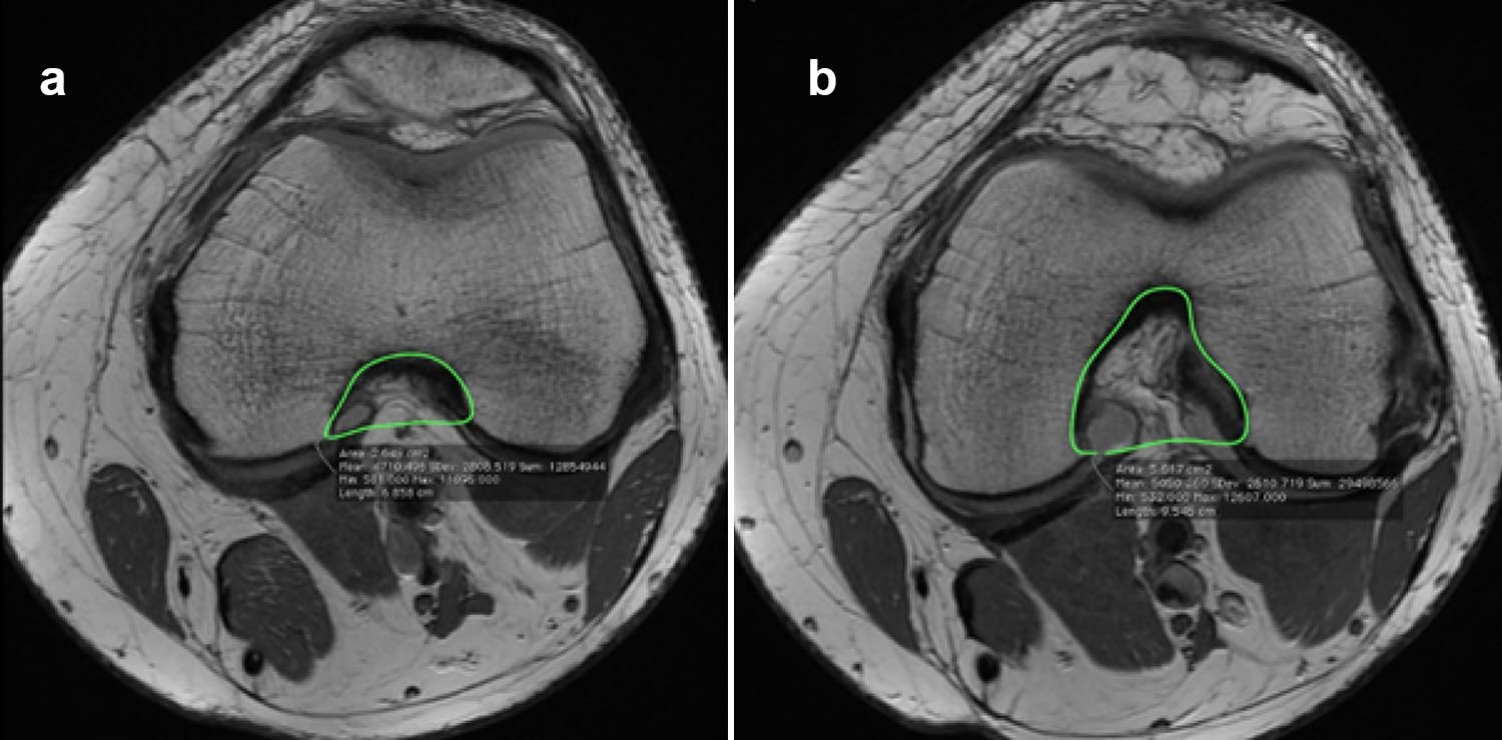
Axial MRI image of the knee, A most proximal, B most distal of the intercondylar notch
